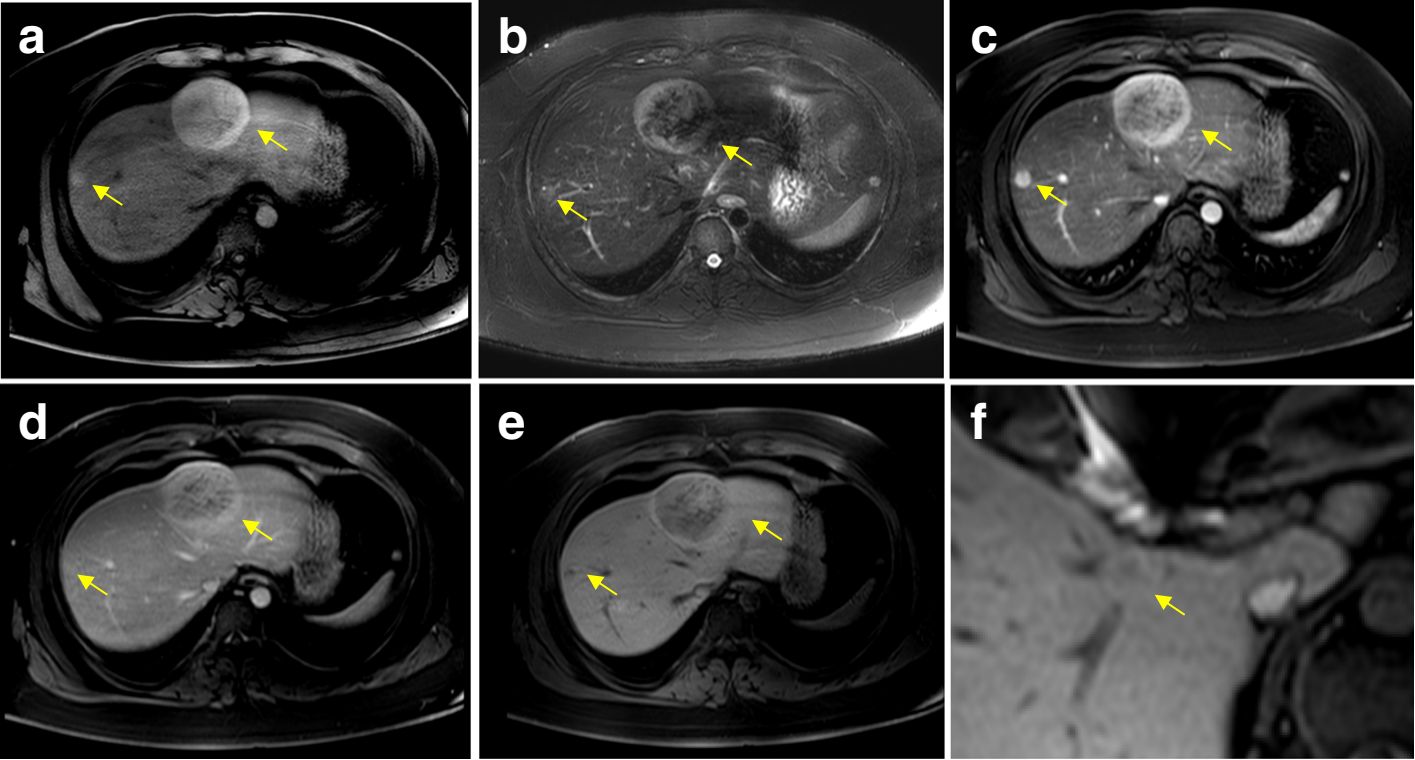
Preoperative magnetic resonance imaging showing the presence of tumors. a T1-weighted magnetic resonance image (MRI) showing the two hyperintense tumors. b T2-weighted MRI showing the two tumors with mild hypertensity. c Gadoxetic acid-enhanced (EOB)-MRI showing the two tumors with enhancement in the arterial phase. d EOB-MRI showing the two tumors with prolonged partial enhancement in the late phase. e EOB-MRI showing the two tumors in segments 3/4 and 8 with low signal intensity in the hepatocellular phase. f EOB-MRI showing the one tumor in segment 1 with low signal intensity in the hepatocellular phase
